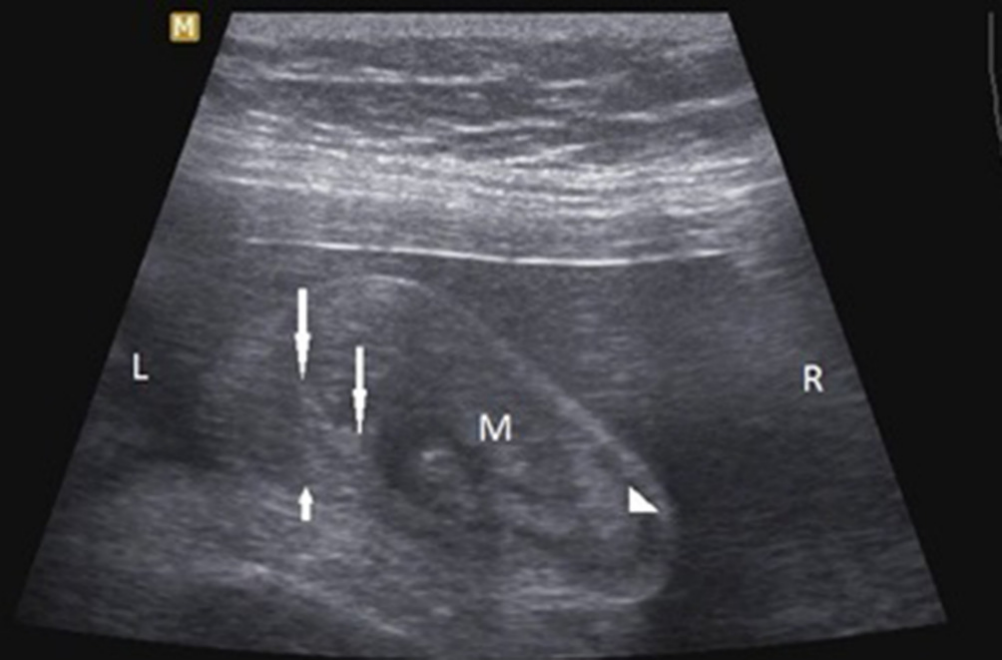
Longitudinal sonography of foetal scrotum showing a right-sided heterogeneous scrotal mass (M) and the displaced right testis (arrowhead). The median raphe (long arrows) is displaced to the left. The left testis (short arrow) is normal.
